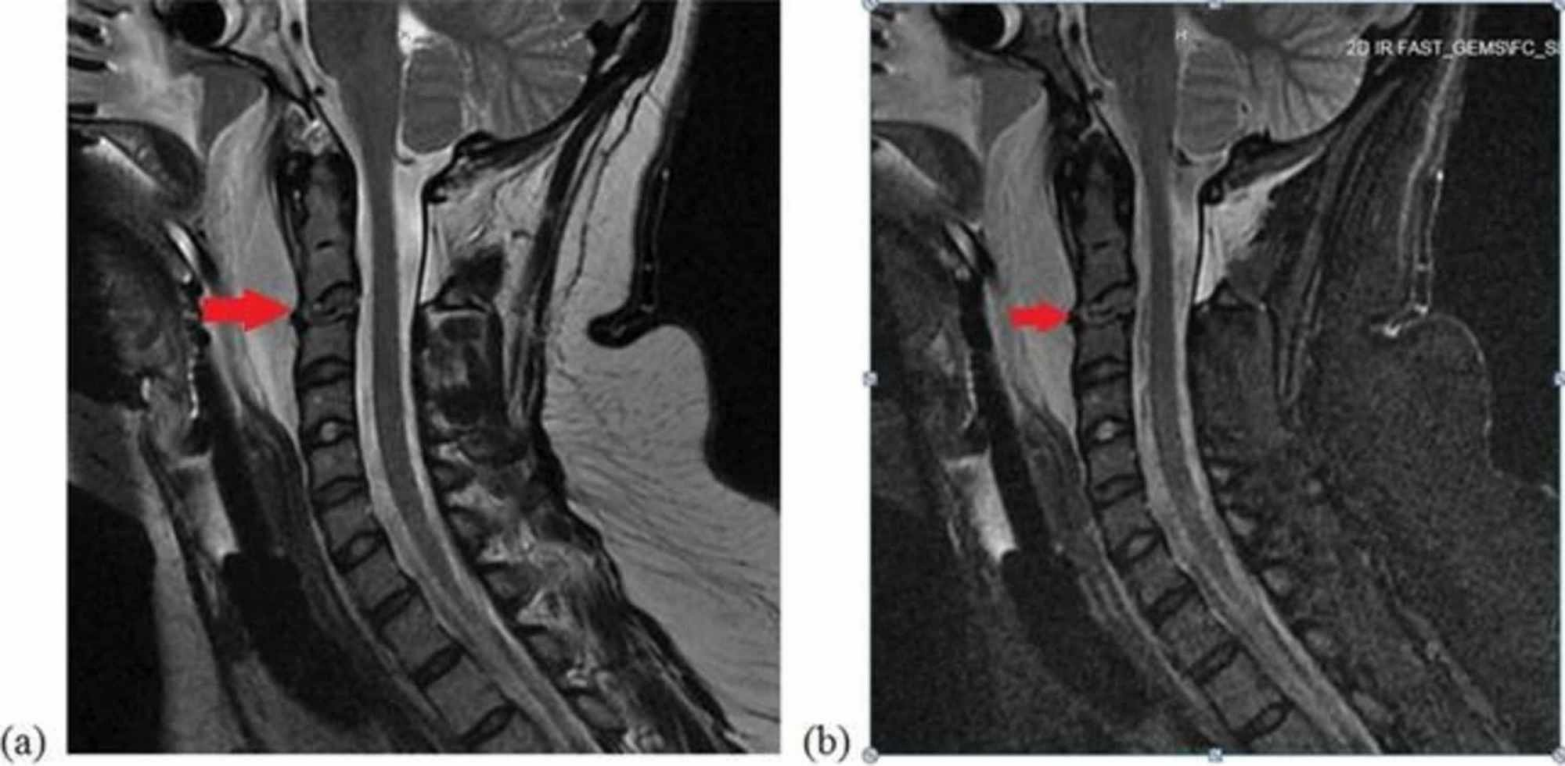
MRI of the cervical spine. (a) T2-Weighted sagittal view and (b) STIR sagittal view of the cervical spine with red arrows show C2-3 intervertebral disc injury.
